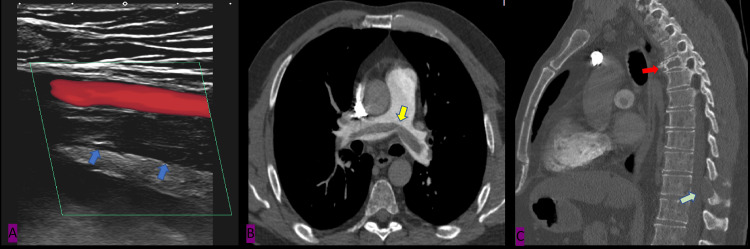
(A) Sagittal lower limb color doppler US shows thrombosed superficial femoral vein (blue arrow) extending to posterior tibial vein (Not shown), (B) Axial CT pulmonary angiogram demonstrates saddle pulmonary embolism (Yellow arrow), (C) Sagittal CT chest with bone reformat demonstrates T4 lytic lesion with compression fracture (Red arrow) and T10 posterior element lytic lesion (Green arrow) and multiple sternal lytic lesions.
